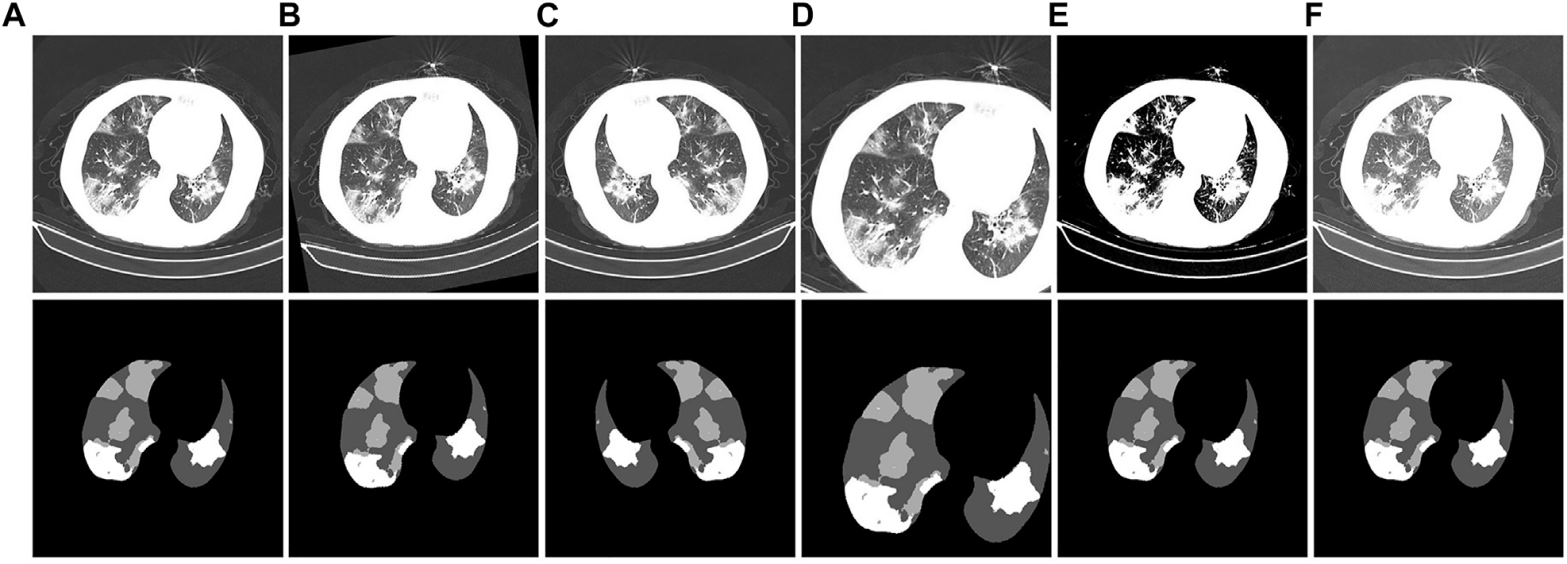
Example of CT image data-enhancement results. (A): the original CT image and its corresponding gold standard. (B): the CT image obtained after rotation and its corresponding gold standard. (C): the CT image obtained after horizontal flipping and its corresponding gold standard. (D): the CT image obtained after random cropping and its corresponding gold standard. (E): the CT image obtained after adjusting the contrast and its corresponding gold standard. (F): the CT image obtained after adjusting the brightness and its corresponding gold standard.
